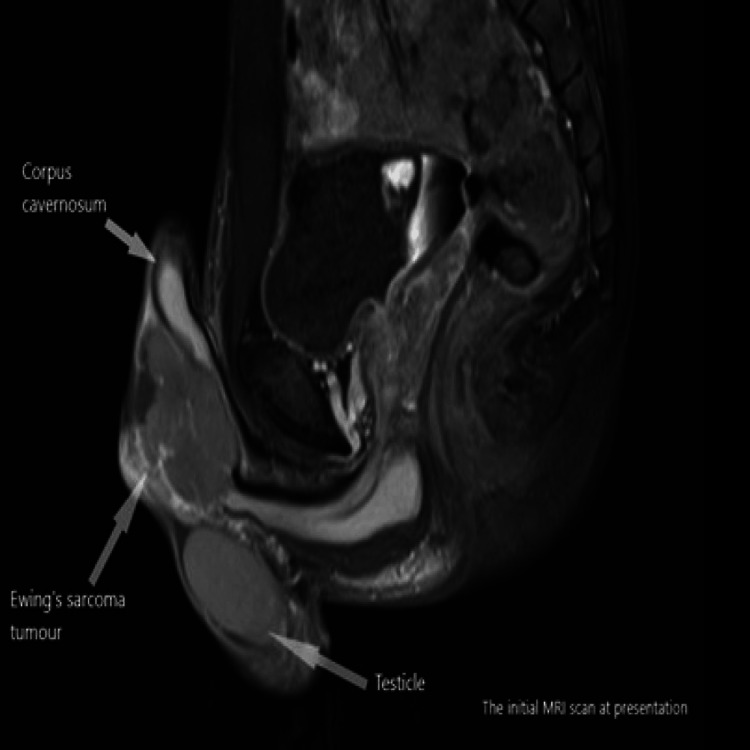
Initial Magnetic Resonance Imaging (MRI) scan at presentation (sagittal plane).
